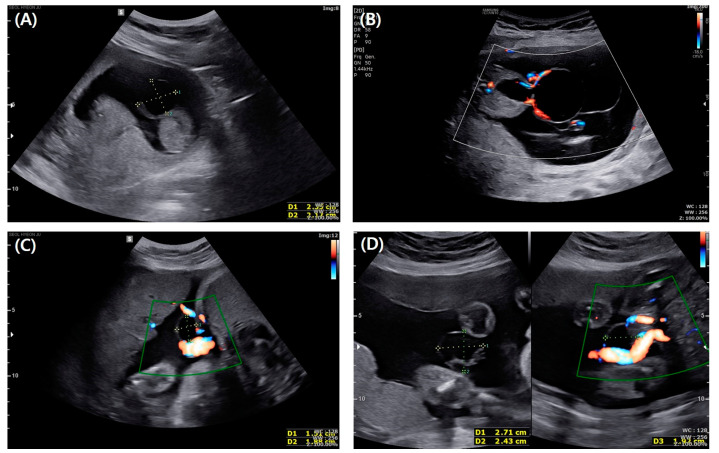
(A) Sonographic finding of the urachal cyst at the gestational age of 13 + 4 weeks shows a 2.4 cm well–defined oval–shaped anechoic cyst located at the proximal part of the umbilical cord. (B) Sonographic finding of the urachal cyst at the gestational age of 17 + 4 weeks shows the size of the cyst increased to 5.4 cm, with a newly appeared 3 cm–sized cyst inside the initial cyst. (C) Sonographic finding of the urachal cyst at the gestational age of 25 weeks shows the size of the cyst has decreased to 2.7 cm. (D) Sonographic finding of the urachal cyst at the gestational age of 32 weeks shows it became 1.8 cm in size.
